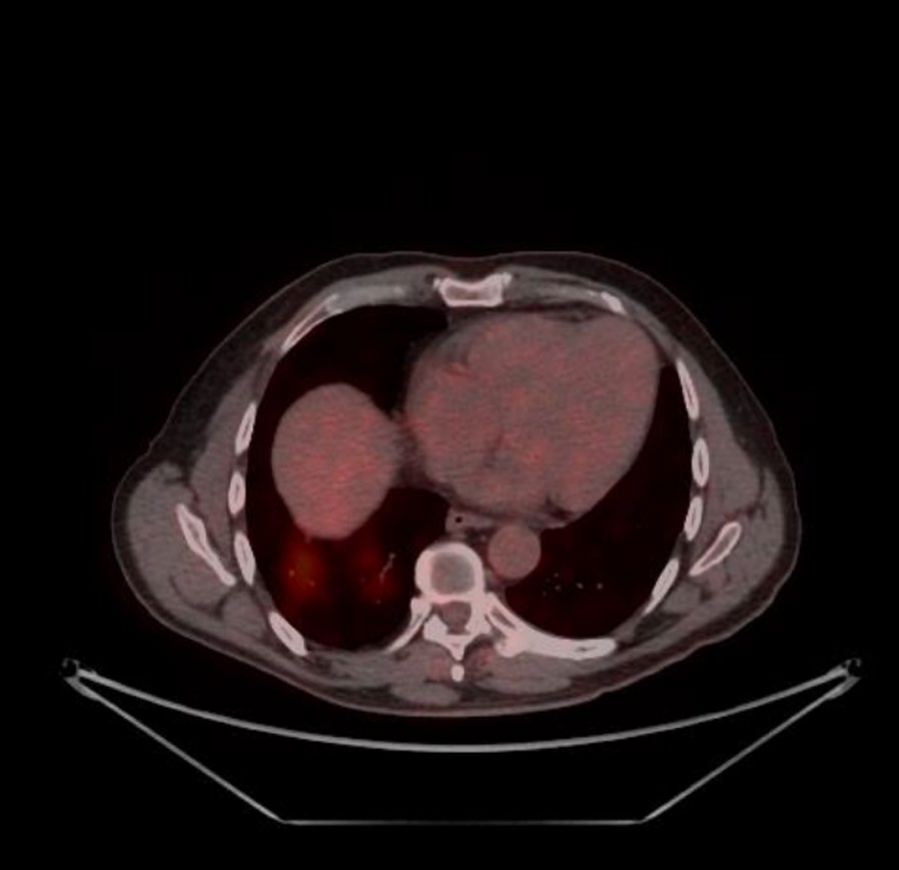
PET-CT 29/04: appearance of multiple areas of discrete opacity bilateral in pulmonary parenchyma, predominating in the base (SUVmax: 3.7-right base. The comparative analysis with a previous examination of 03/18/2020 shows: the degree of glycolytic hypermetabolism persists without significant variation associated with reduced dimensions, in heterogeneous tissue formation. The findings are compatible with uptake above mediastinal, but below or equal to uptake in the liver with nodal or extranodal sites with or without a residual mass indicating a non-progressive disease with a complete metabolic response to lymphoma treatment. The appearance of multiple areas of ground-glass opacities sparse by the bilateral pulmonary parenchyma, predominantly at the right base, suspicious for viral pneumonia
